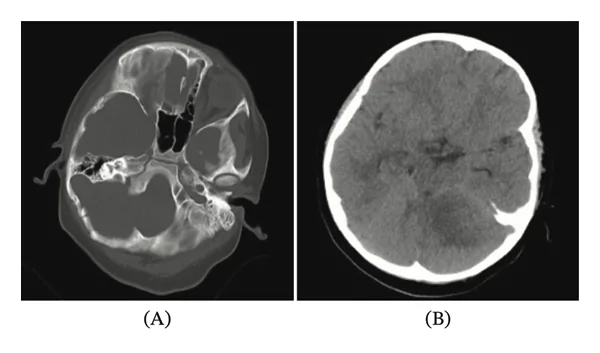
CT head without IV contrast. (A, B) Mass within the left cerebellar hemisphere with associated vasogenic edema. There is no associated herniation or hydrocephalus.
